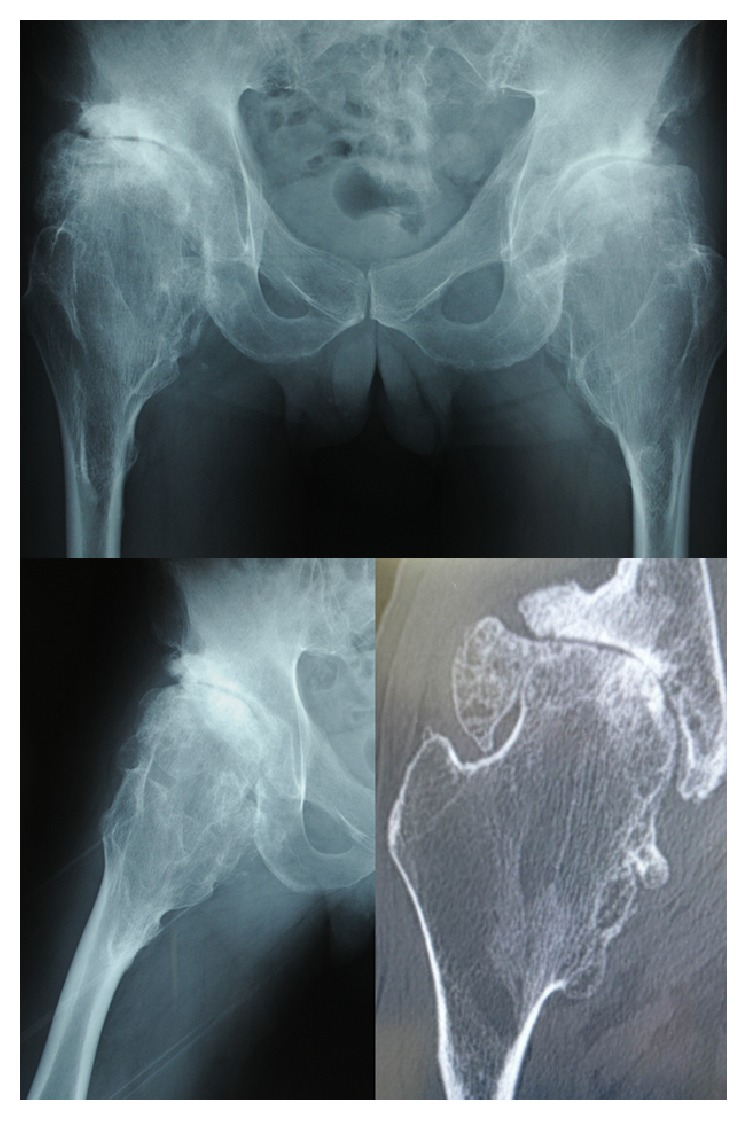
Preoperative radiographs and multidirectional computed tomography image of the right hip joint showing the gigantic femoral head, increasing angle of the neck shaft, and large diameter of the femoral neck.
